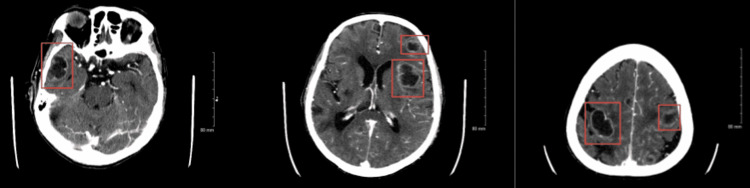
CT scan of the head with contrast showing multiple ring-enhancing lesions suggestive of neoplasm/malignancy.
